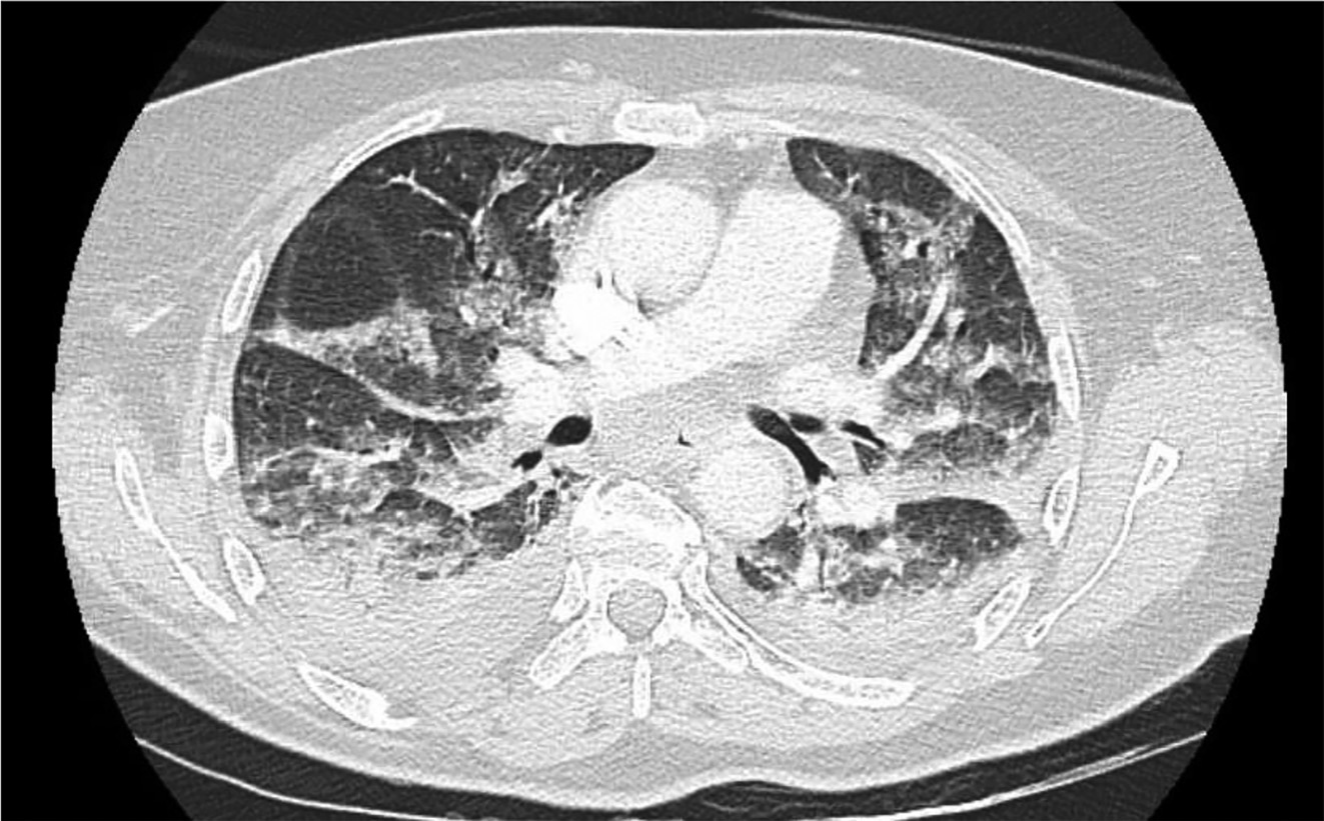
CT pulmonary angiography and thoracic–abdominal–pelvic computed tomography during respiratory failure with no pulmonary embolism, no sign of post PIPAC complications in the abdomen but bilateral pleural diffusion with passive atelectasis and alveolar–interstitial syndrome.
